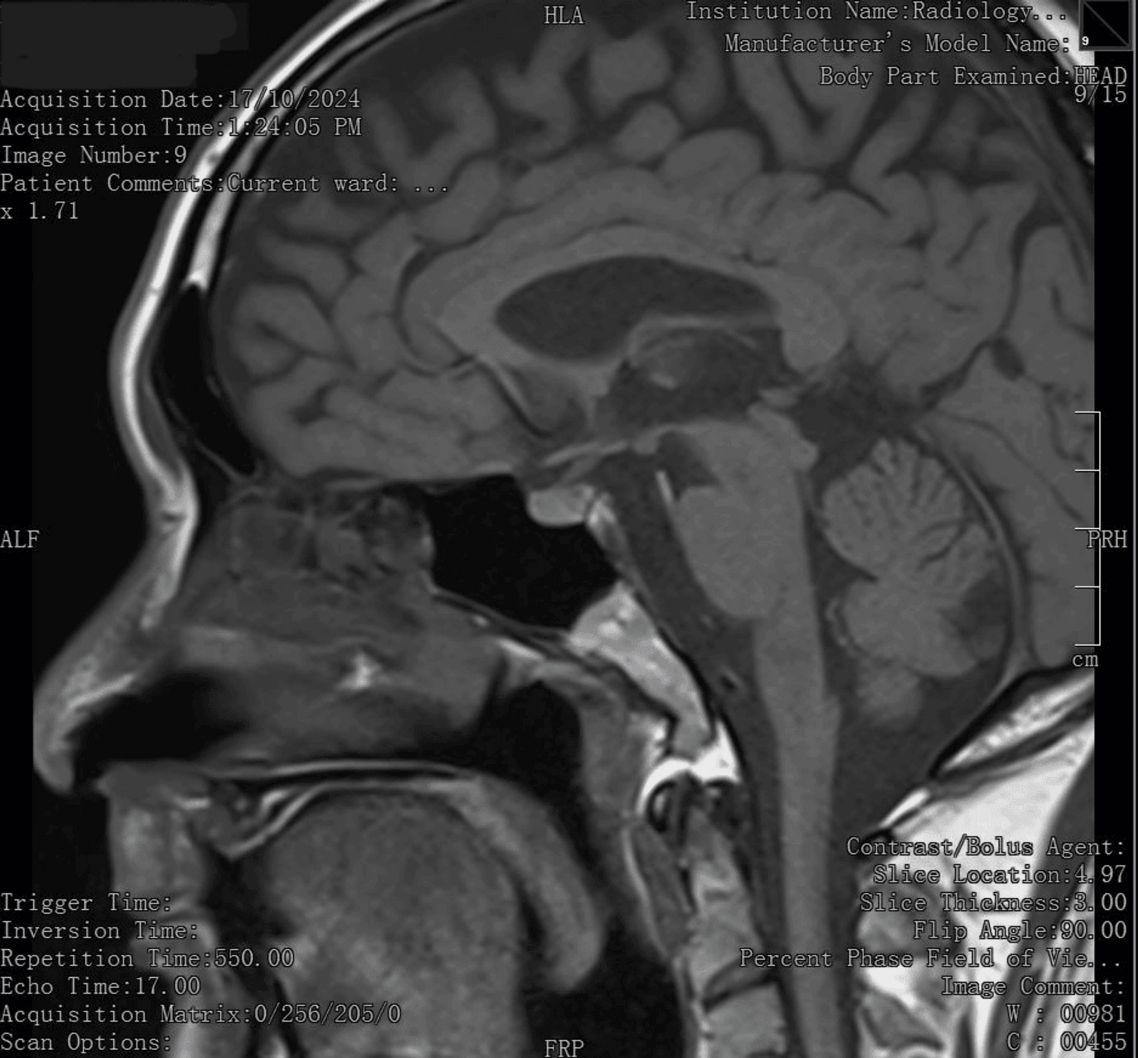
Magnetic resonance imaging of the brain (sagittal view) depicted no lesions at the pituitary.
